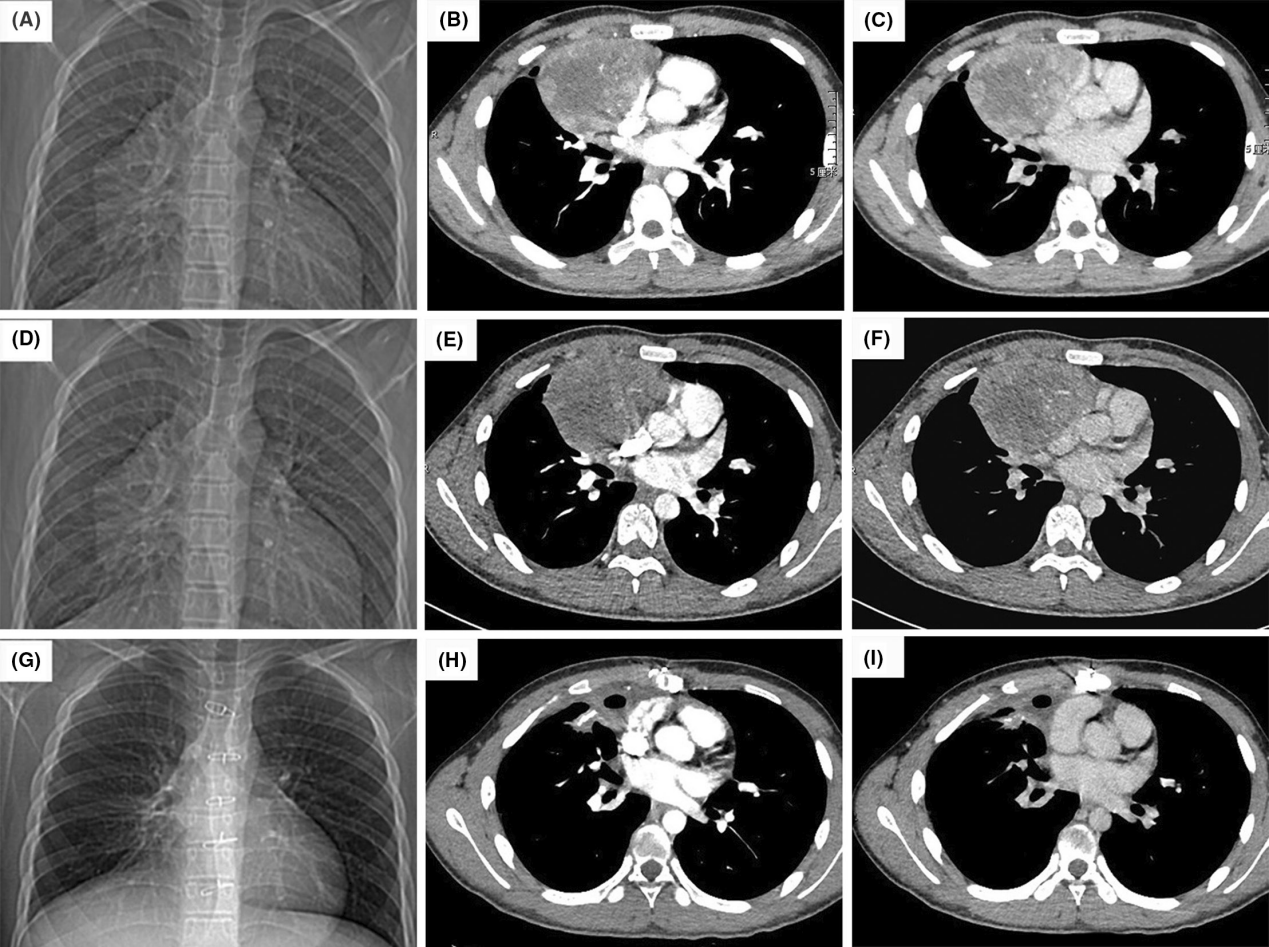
(A) Anterior–posterior chest x‐ray and (B, C) chest computed tomography scans of arterial and venous phase: a huge tumor of mediastinum before treatment; (D) anterior–posterior chest x‐ray and (E, F) chest computed tomography scans of arterial and venous phase: the size of the tumor was not significantly changed after 6 cycles of chemotherapy; (G) anterior–posterior chest x‐ray and (H, I) chest computed tomography scans of arterial and venous phase: no sign of tumor recurrence 6 months after surgery.
